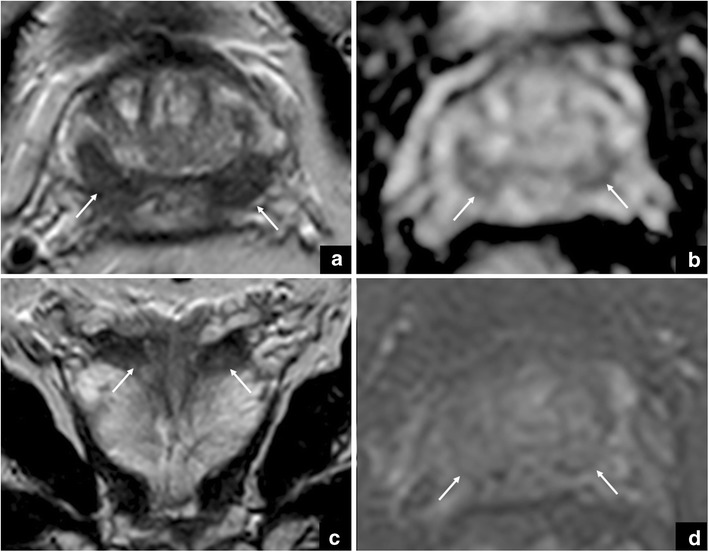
The arrows show two median, symmetric, bilateral areas of low signal intensity on axial (a) and coronal (c) T2-weighted imaging, with restricted diffusion in the ADC map (b) and diffuse enhancement on DCE imaging (e). This set of appearances has been called the “*moustache sign*”
